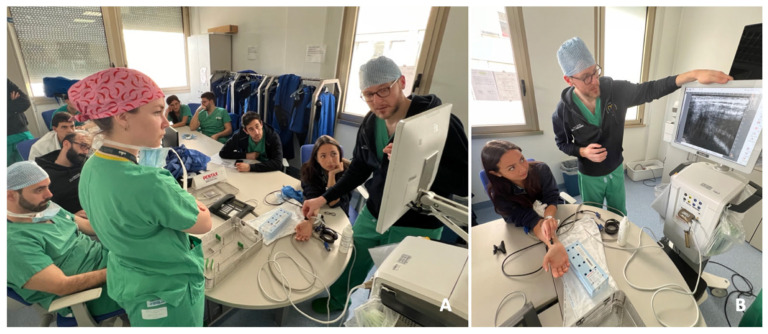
Cardiac surgery residents’ training: (A) Before the start of the study, all residents received a theoretical and practical training session on transit-time flow measurement and high-resolution epicardial ultrasonography, conducted by the benchmark surgeon. (B) In addition, residents were given the opportunity for an additional hands-on session, during which they practiced epicardial ultrasonography on their own radial artery.
